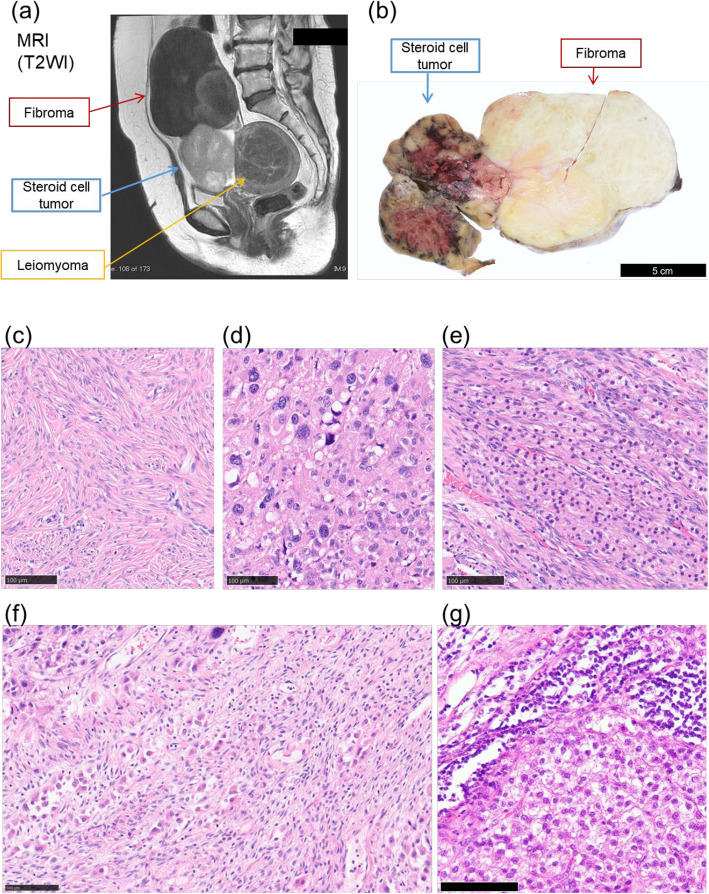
Radiological and pathological images of ovarian tumors. a Sagittal T2-weighted pelvic magnetic resonance imaging (MRI). The upper tumor with low signal intensity, middle tumor with high signal intensity, and right bottom tumor in the uterus is a fibroma, steroid cell tumor (SCT), and leiomyoma, respectively. b Macroscopically, the two tumors coexist. The fibroma is a white firm mass, and the SCT is a yellow tumor with bleeding and necrosis. Bar=5 cm. A high-power view of (c) fibroma and (d, e) SCT. d Most tumor cells show significant nuclear atypia. e Some tumor cells have small, round nuclei with less atypia. f Fibroma and SCT cells are intermingled in the boundary between the two tumor components. g Lymph node metastasis of the SCT. Metastatic tumor comprises steroid tumor cells with less atypia, similar to Figure 1e. c-g Bar=100 mm
